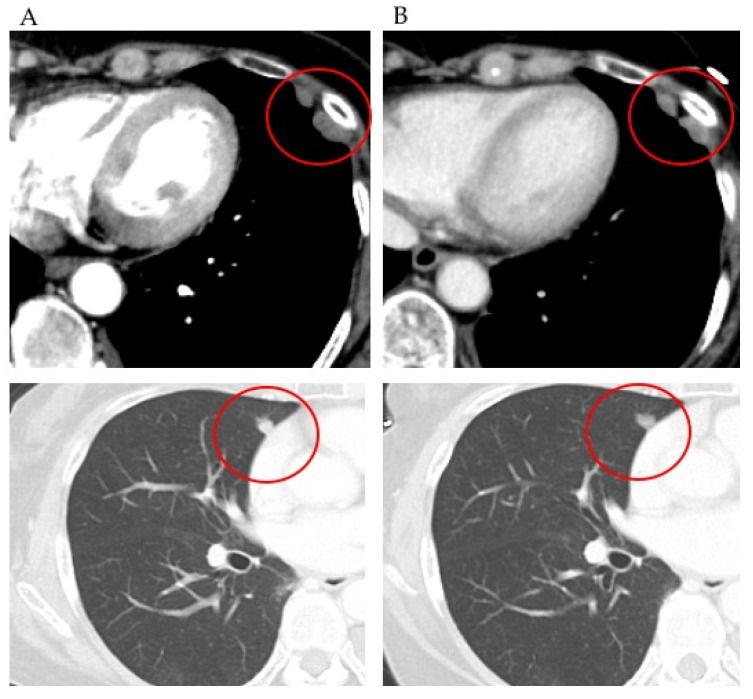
(A) Chest computed tomography showing a lung metastatic lesion before everolimus treatment. (B) Chest computed tomography showing a shrunken lung metastatic lesion, treated with everolimus, at the end of the first month.
